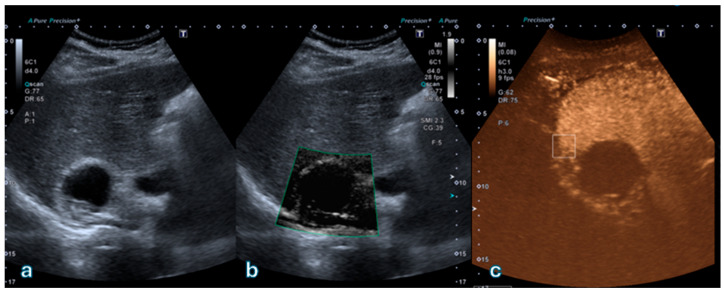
Complex cystic lesion located in the upper pole of the right kidney. (a) B-mode ultrasound shows a cyst with a dependent hyperechoic intracystic component movable with changes in patient position, suggesting proteinaceous or hemorrhagic material rather than a solid nodule. (b) Superb Microvascular Imaging (SMI) demonstrates no detectable vascular signal within the hyperechoic component or cyst wall. (c) Corresponding contrast-enhanced ultrasound (CEUS) image confirms absence of enhancement, consistent with a proteinaceous cyst classified as Bosniak II. This case exemplifies concordant findings between SMI and CEUS in a Bosniak II lesion.
